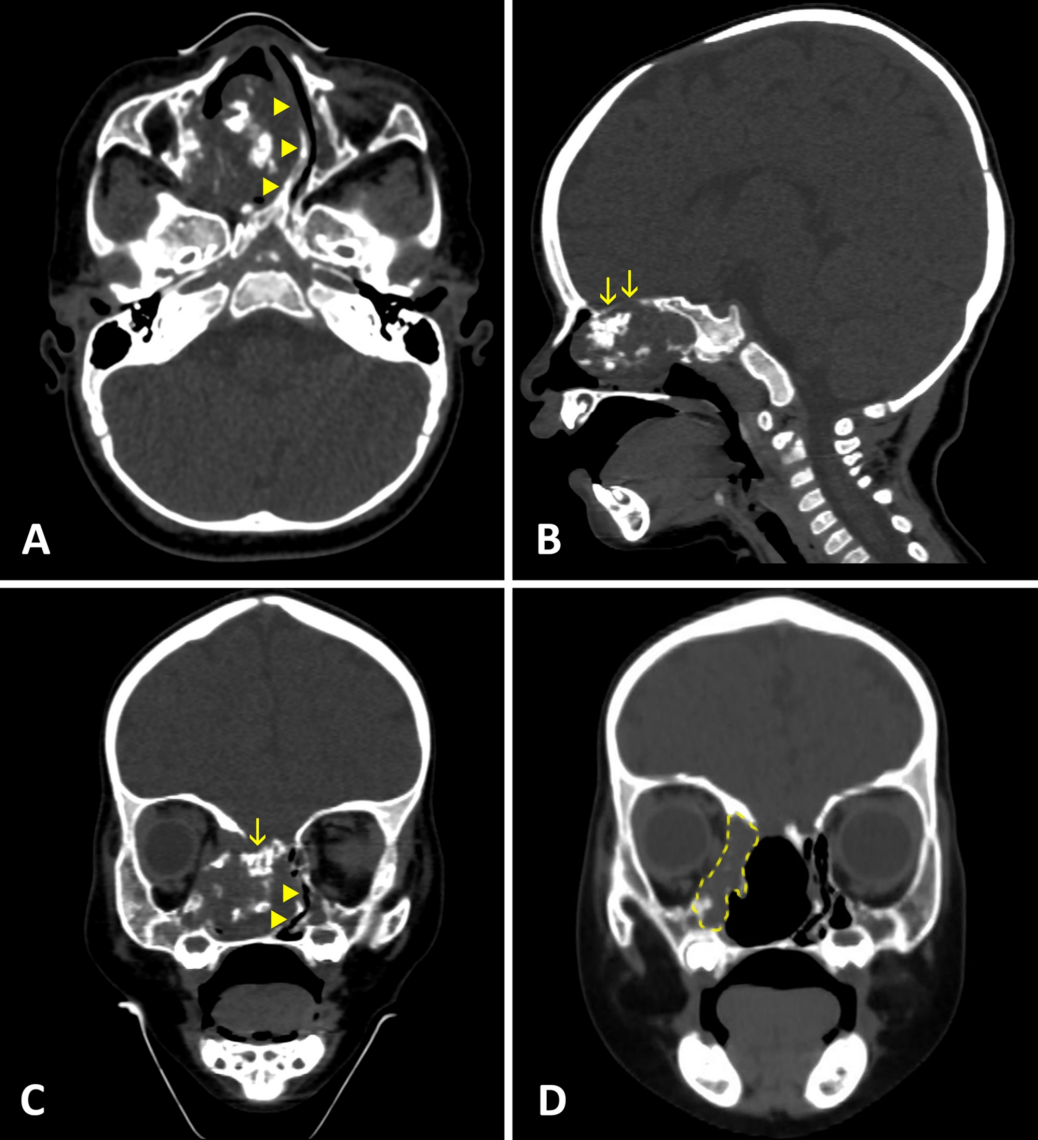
CT scans before (A-C) and after (D) the first surgery The lesion occupies the right nasal cavity, ethmoid labyrinth, maxilla with limited orbital, and skull base extension (arrows). Calcifications are visible within the tumor mass. Displaced nasal septum is marked by arrowheads. Postoperative coronal CT scan (D) shows unresected lateral portion of the tumor.
